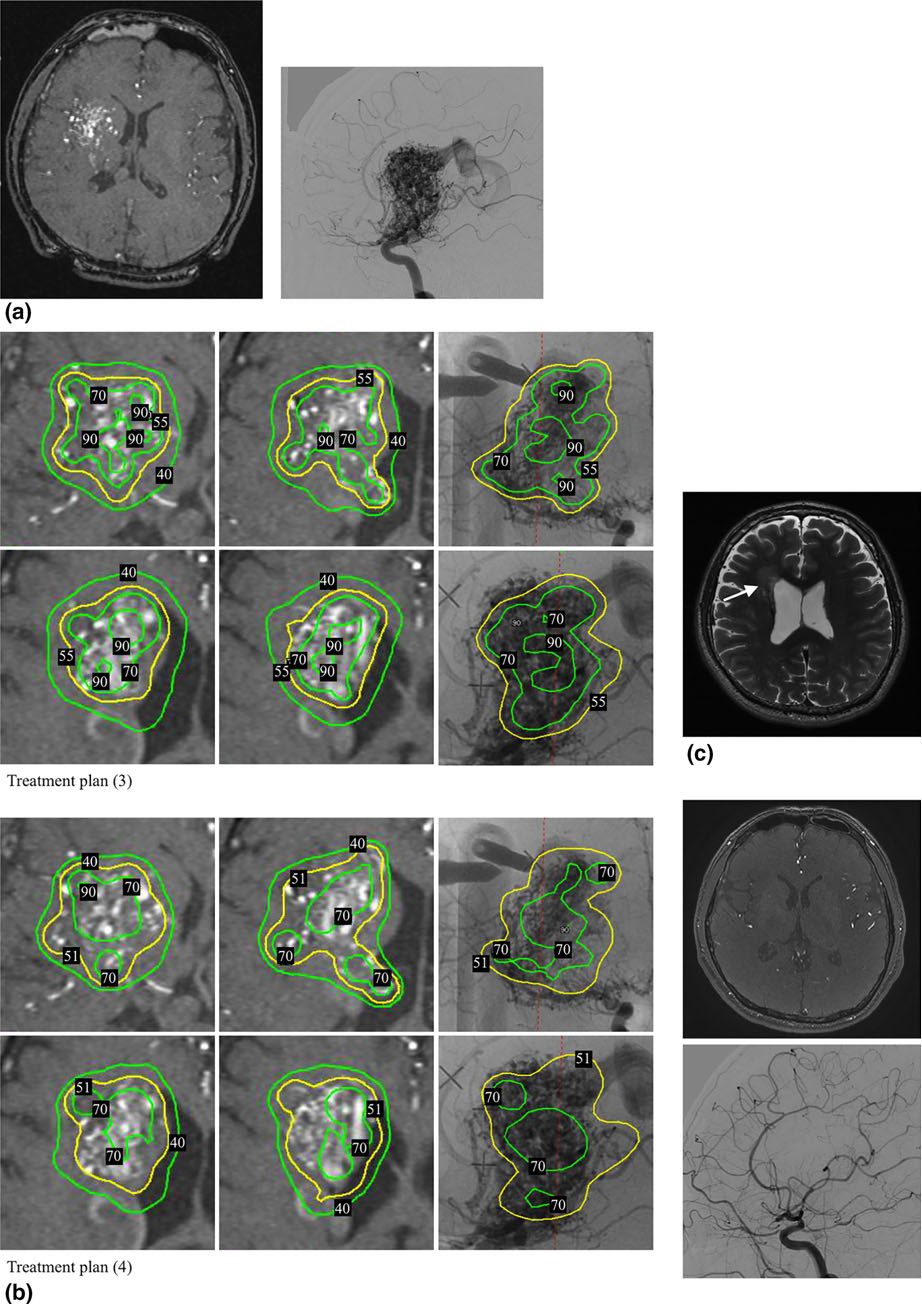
(a) An AVM with a volume of 26.9 cm^3^ was treated by GKRS. (b) Comparison of two treatment plans for this AVM, plan (3) with the normalization effect reduction and plan (4) without the normalization effect reduction. The AVM was treated by using plan (3). (c) Follow‐up T2 MRI 12 months after treatment revealed a partial regression of the AVM nidus with temporary and mild radiation‐induced edema (white arrow), but had no neurological symptoms. (d) Follow‐up TOF MRI and cerebral angiogram 19 months after treatment revealed a complete obliteration of the AVM.
